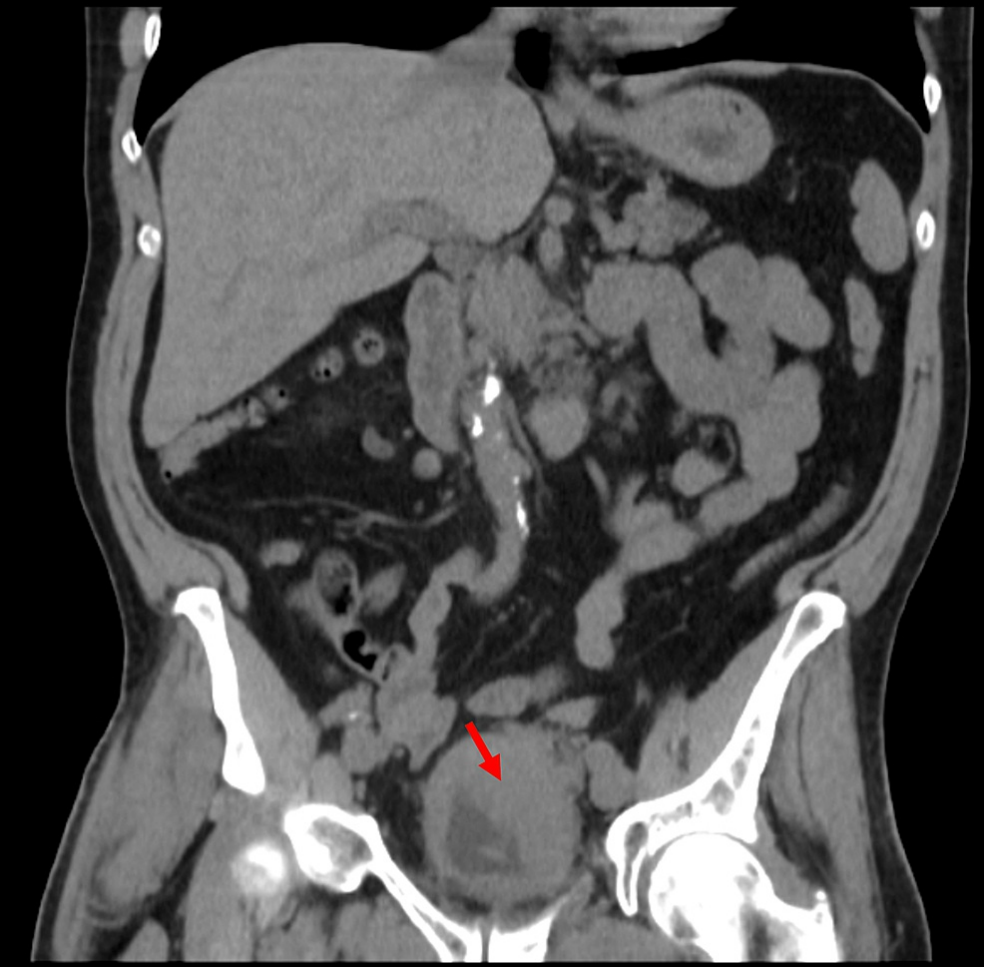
Coronal CT Findings This non-contrast CT scan from a coronal perspective reveals an infiltrating left-sided bladder tumor (highlighted by red arrow), lymphadenopathy, and fat infiltration.
